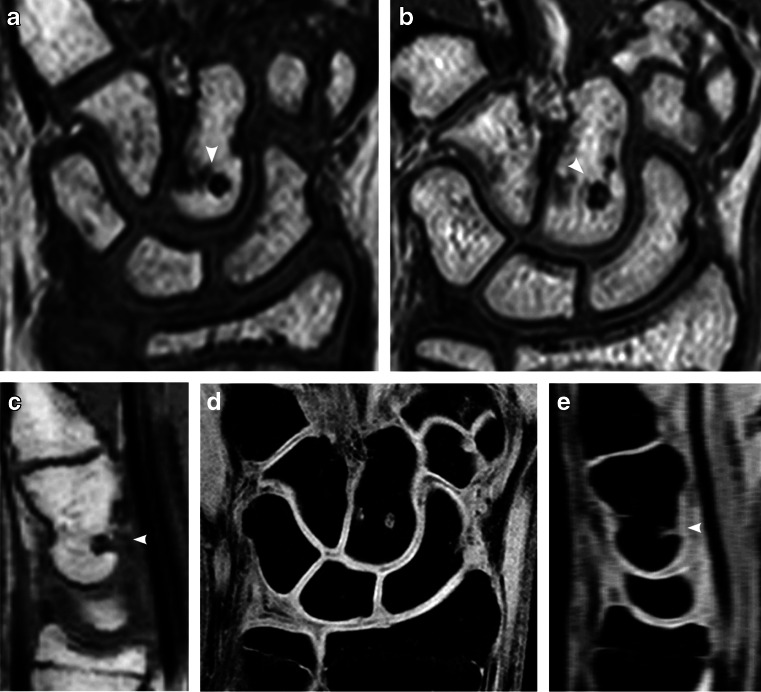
Depression (*arrowheads*) on the volar side of the capitate bone. a–c T1-weighted turbo spin-echo MRI in a coronal plane in an 11-year-old boy (a) and unchanged at 4-year follow-up seen on coronal image (b) and, better, in a sagittal reconstruction (c). d, e On the water-selective (volumetric steady-state spoiled gradient echo) cartilage sequence at 4-year follow-up, the signal within and covering the depression is lower than that of the articular cartilage, as seen in a coronal image (d) and, better, in a sagittal reconstruction (e), suggesting it is not covered
